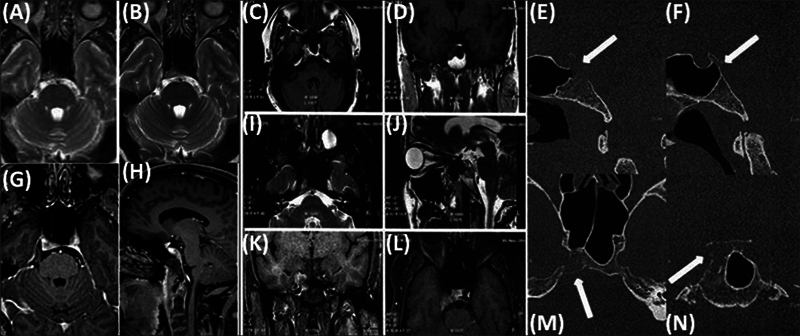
Post-gadolinium fat-saturated T1-weighted brain magnetic resonance images, axial (
